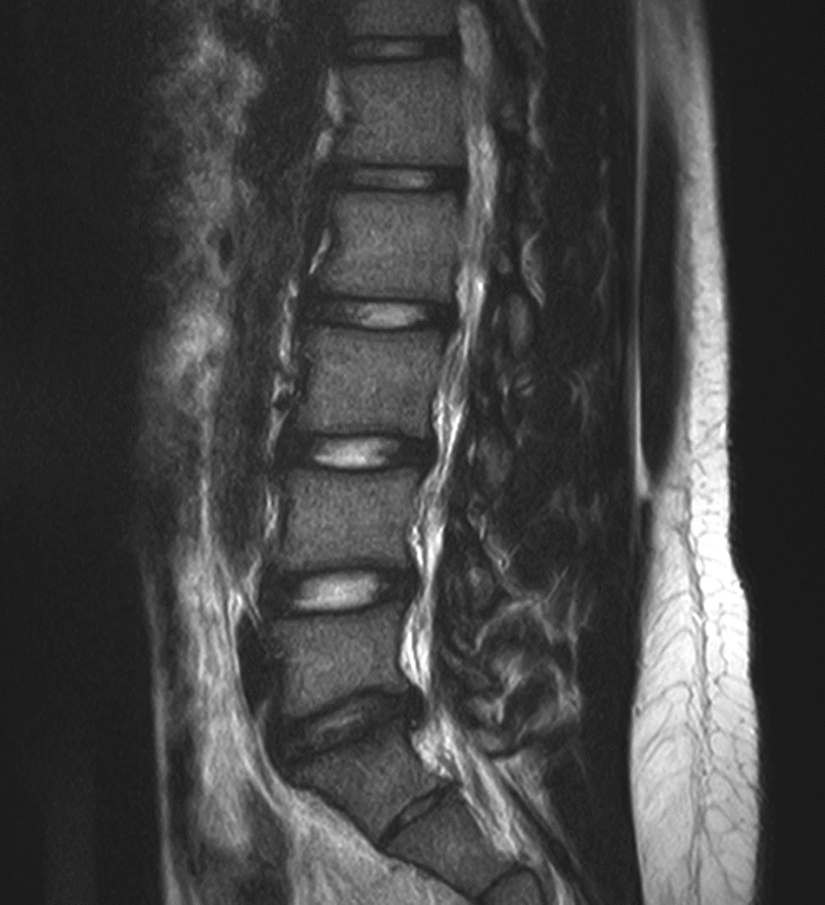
MRI obtained at the boy’s 40-month follow-up showed no significant changes of platyspondyly of the lumbar vertebrae and no significant resorption of the herniated L5/S1 lumbar disc
